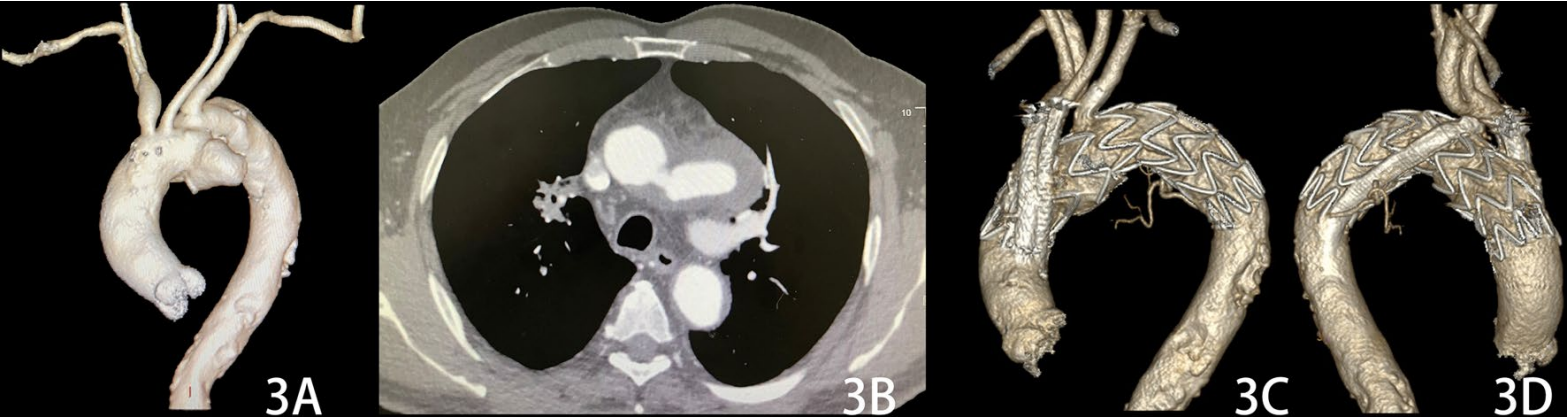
A, B The CT angiography of case 16 showed a pseudoaneurysm located at the aortic arch, and the patient underwent the triple chimney technique. C, D, CT angiography showed patent chimney stent grafts 18 months after the operation
